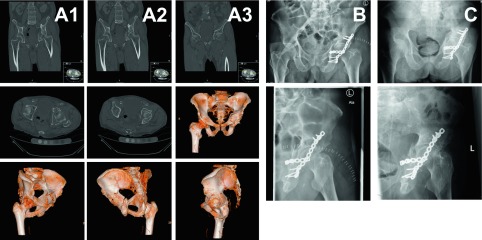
Two-column fracture of the left acetabulum following a bicycle accident in a 76-year-old male. Significant premorbidities included cardiomyopathy with s/p myocardial infarction nine years ago, recurrent DVTs with warfarin anticoagulation, lower limb atherosclerosis with s/p femoro-popliteal bypass, and s/p viral encephalitis with residual impaired gait and presence of suprapubic catheter. Due to s/p multiple abdominal surgeries, the anterior approach was no option. The individualized treatment plan was defined as ORIF via one singular Kocher-Langenbeck approach, aiming to convert the two-column fracture into a stable fracture pattern with secondary congruence of the hip joint. Computed tomography (CT) scans show the two-column fracture in frontal, axial views and 3D reconstruction (column A1–A3), postoperative conventional radiographs display the reduced joint line at two weeks (column B) and at three months follow-up (column C).
